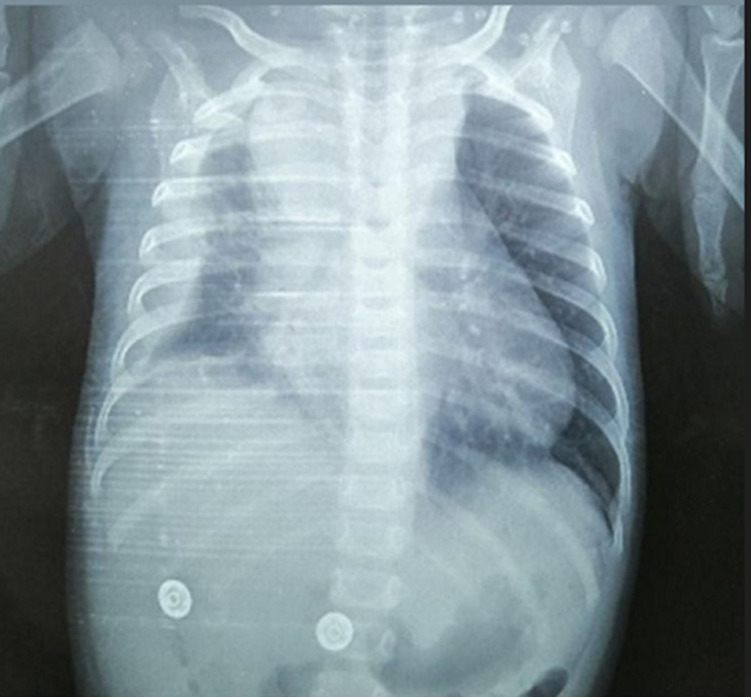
the control chest X-ray after evacuating puncture showing right loculated pleurisy and mediastinal enlargement with a heart pushed back to the left
